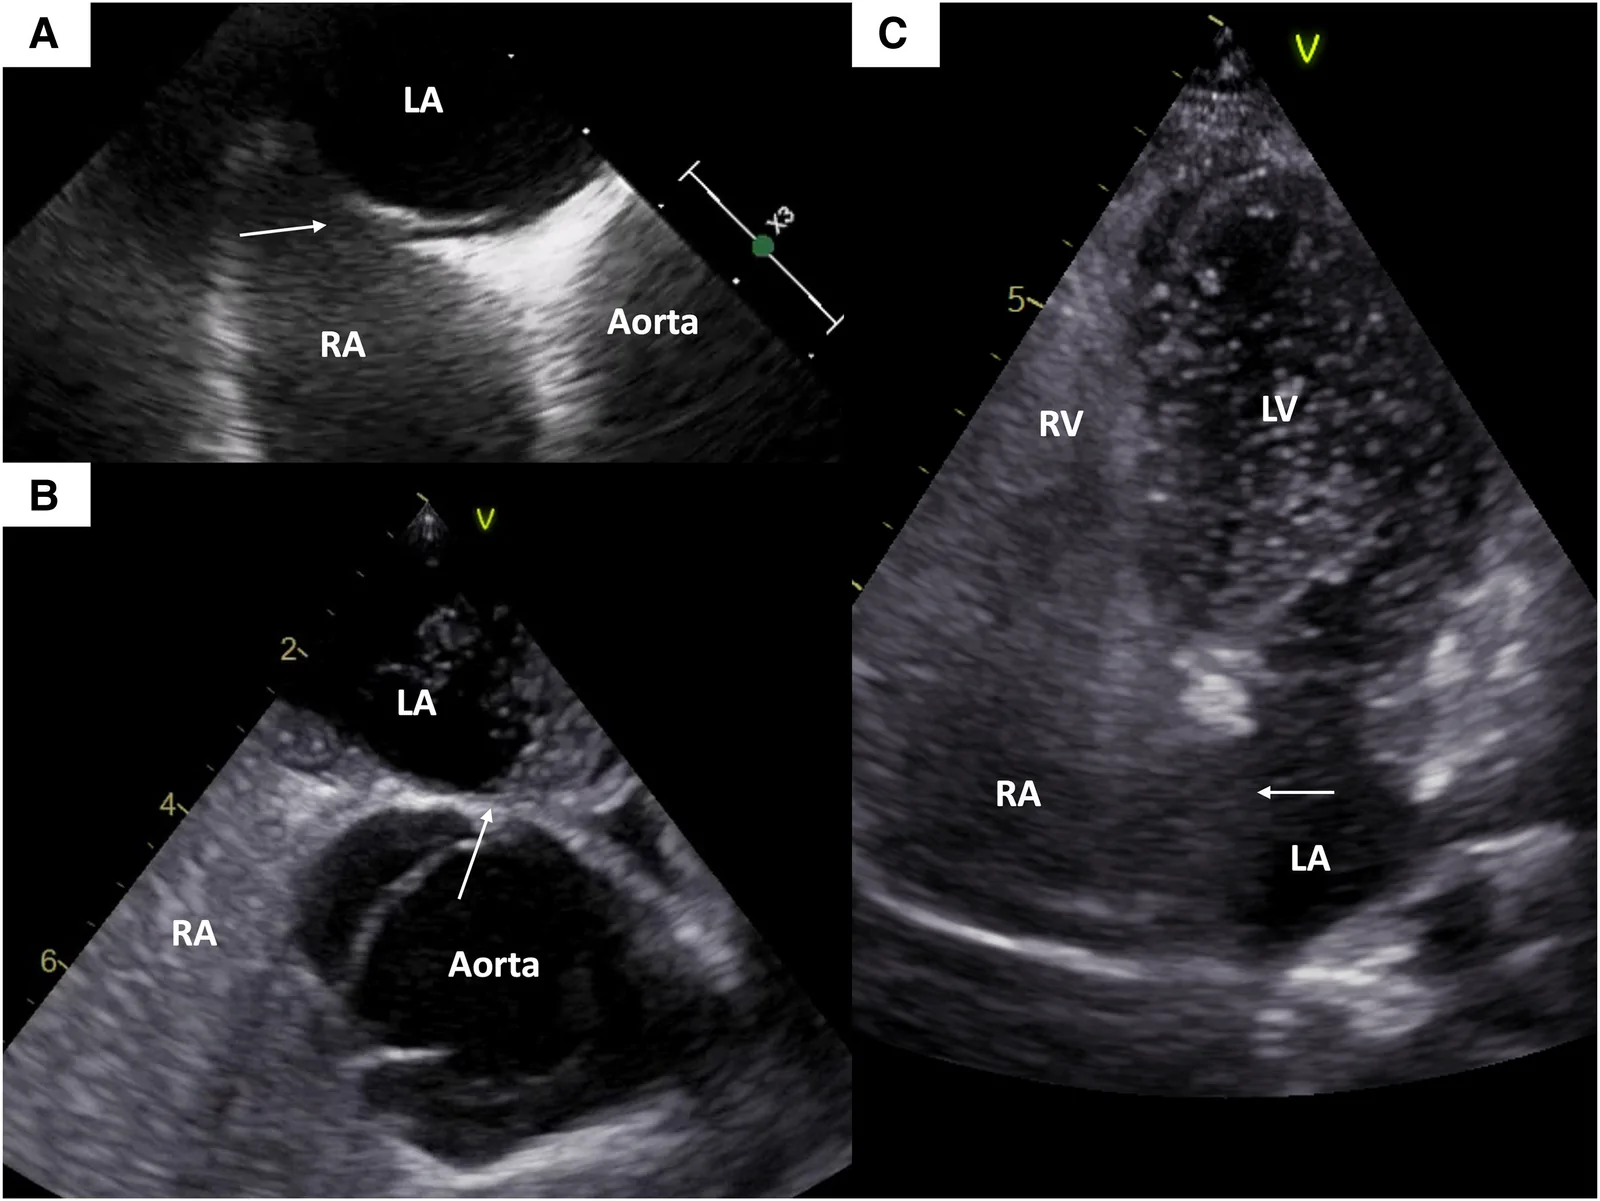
Echocardiographic assessment of patent foramen ovale (PFO) using bubble contrast test. (*A*) Two-dimensional transoesophageal echocardiography (TEE) image demonstrating transient leftward bowing (‘septal bounce’) of the IAS toward the left atrium (LA, arrow) following release of the Valsalva manoeuvre, indicating a pressure gradient favouring right-to-left shunting. (*B*) Contrast-enhanced TEE showing microbubble passage from the right atrium (RA) through a patent foramen ovale into the LA (arrow), confirming the presence of an interatrial communication. (*C*) Transthoracic echocardiography (TTE) images obtained during contrast study demonstrating right-to-left shunt upon Valsalva release, with leftward deviation of the atrial septum (arrow). LA, left atrium; RA, right atrium; LV, left ventricle; RV, right ventricle.
